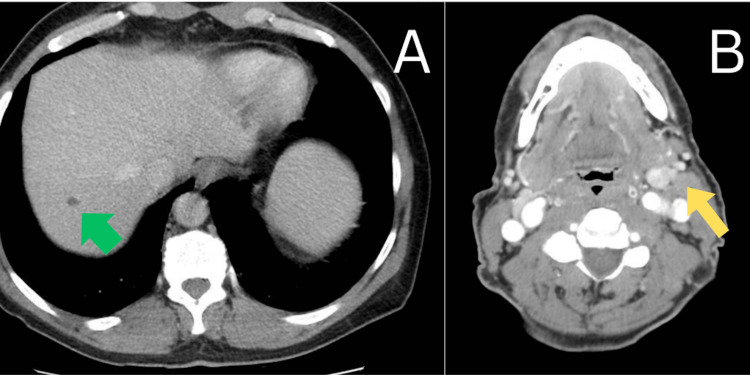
CT scan of the abdomen, pelvis, and neck with contrast. Image A demonstrates one of several indeterminate hypoattenuating lesions in the liver (green arrow). Image B demonstrates an enlarged, 13-mm, level two submandibular lymph node (yellow arrow).
